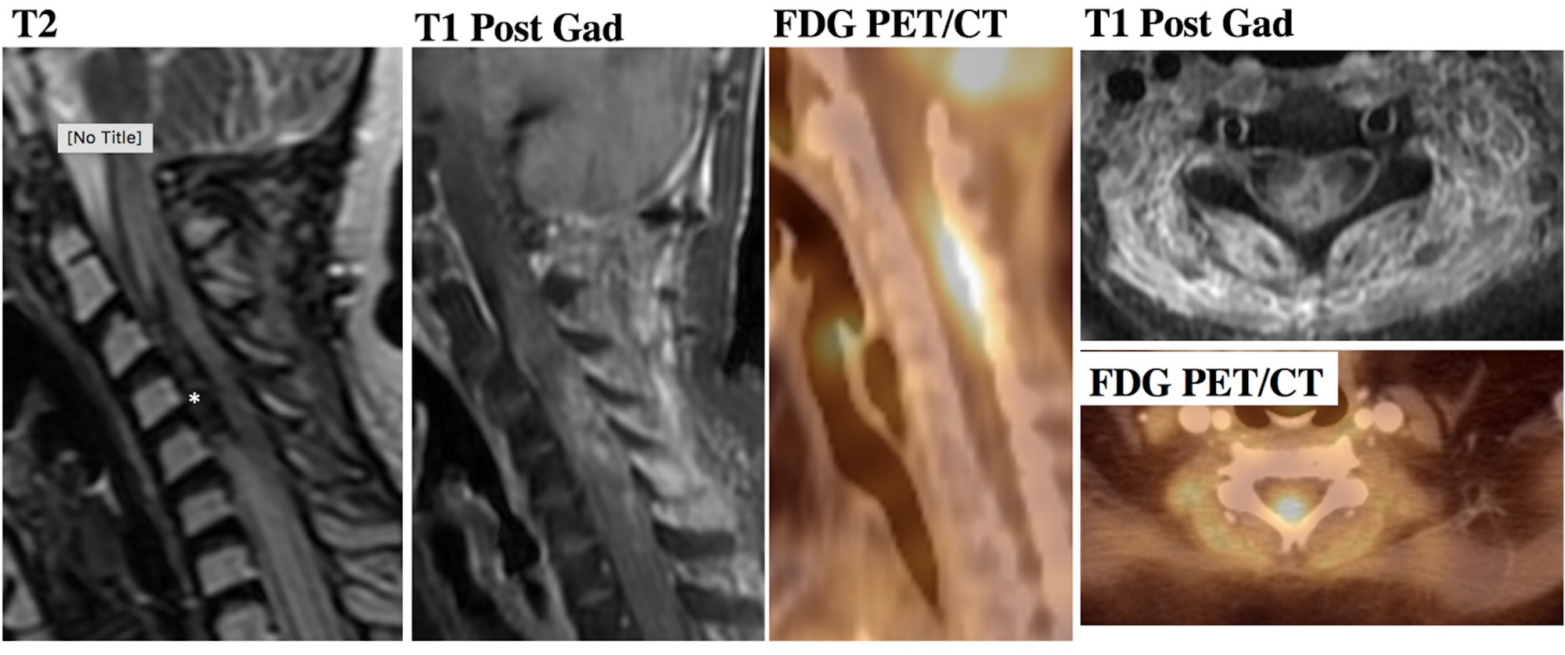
High-grade pineal parenchymal tumor in a patient with disseminated disease to the spine Region of enhancement of the cervical spinal cord spanning from C2 to C7 with evidence of blood products indicated by an asterisk (*). 18F-FDG PET demonstrates avid uptake of the tracer within the enhancing portion of the spinal cord, indicating a recurrent tumor. 18F-FDG PET: 18F-fluorodeoxyglucose positron emission tomography
